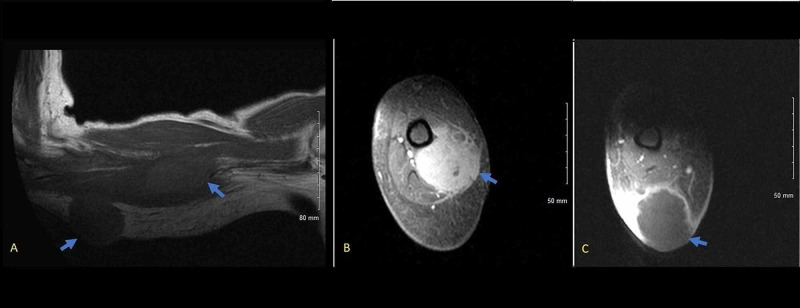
Images of the MRI of left upper extremities. (A) shows both proximal and distal mass in coronal section, (B) shows proximal deep soft tissue mass between biceps and triceps in left arm, (C) shows distal soft tissue subcutaneous mass.
